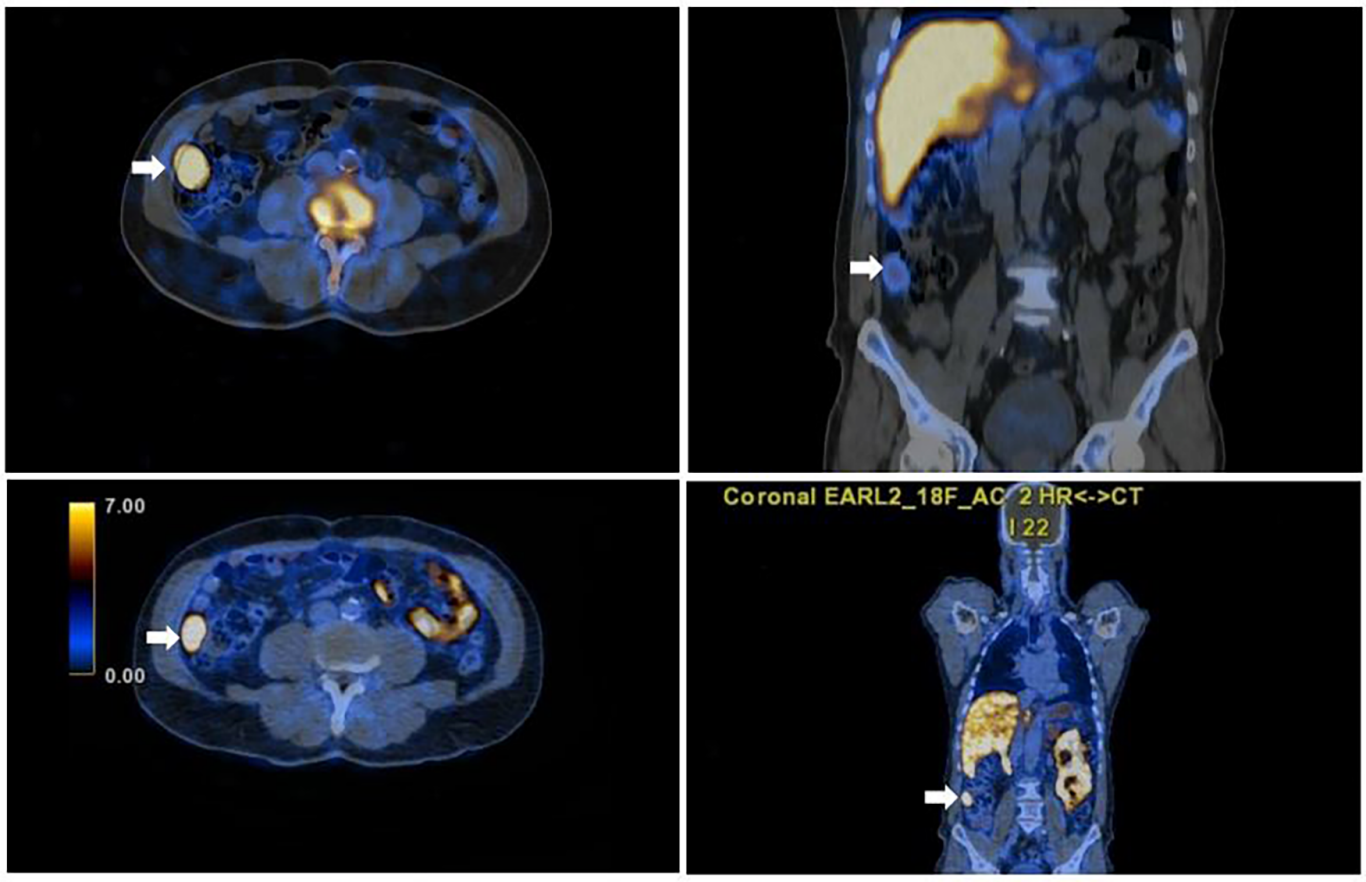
Axial and sagittal fused SPET/CT images of Tc-99m scan (left and right upper images) confirming functioning abdominal splenic tissue and concordant with the focal PSMA uptake seen on the axial and sagittal PSMA PET/CT images (right and left lower images).
